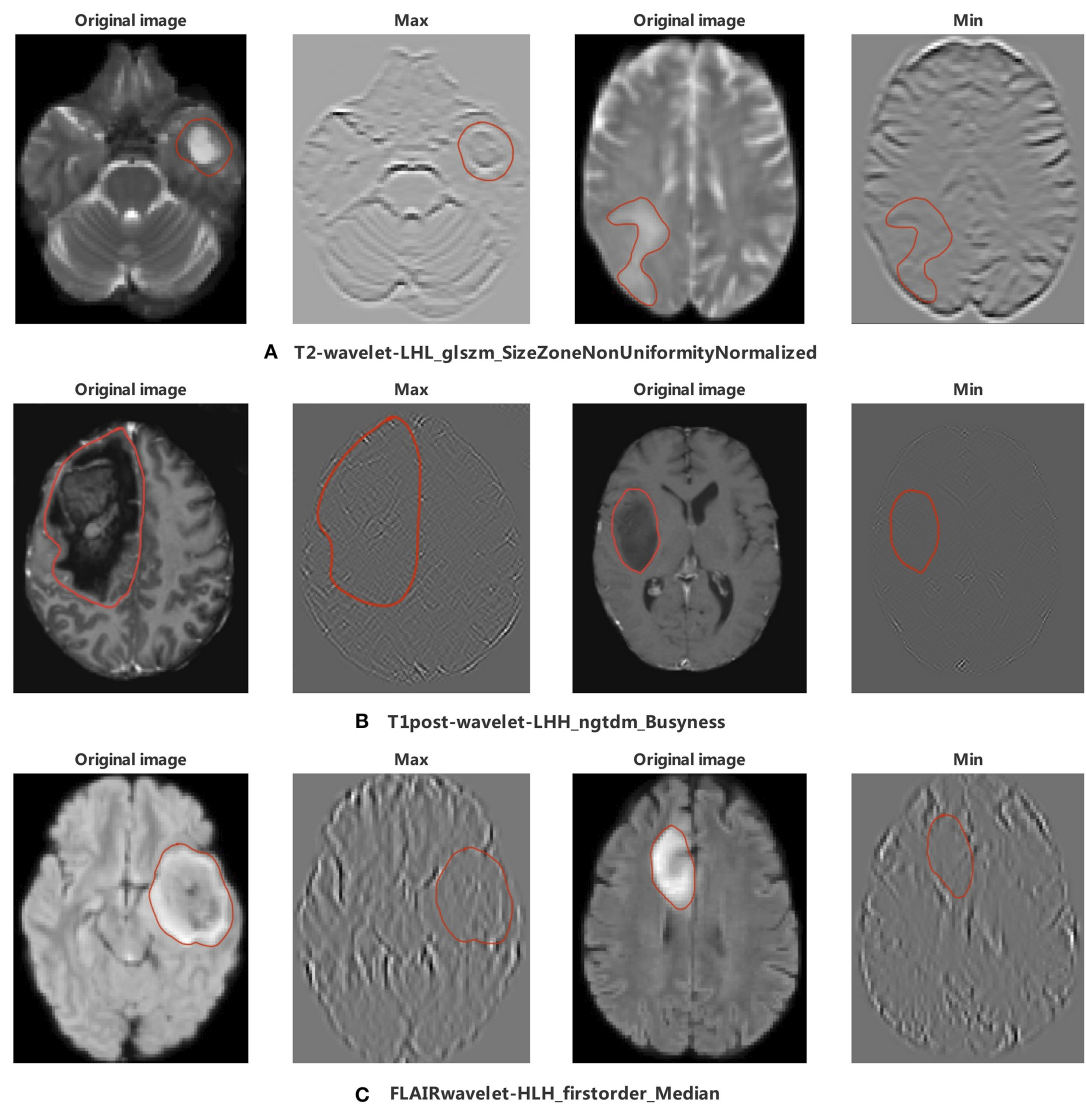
The appearance of the MR image corresponding to the top 3 radiomic features in glioma. The region encircled in red represents the lesion boundary. (A) Images corresponding to the maximum and minimum of T2-wavelet-LHL_glszm_SizeZoneNonUniformityNormalized. (B) First column images are the Wavelet images corresponding to the maximum and minimum value of T1post-wavelet_LHH_ngtdm_Busyness, second column images are original images of wavelet images. (C) Images corresponding to the maximum and minimum value of FLAIR-wavelet-HLH_firstorder_Median.
